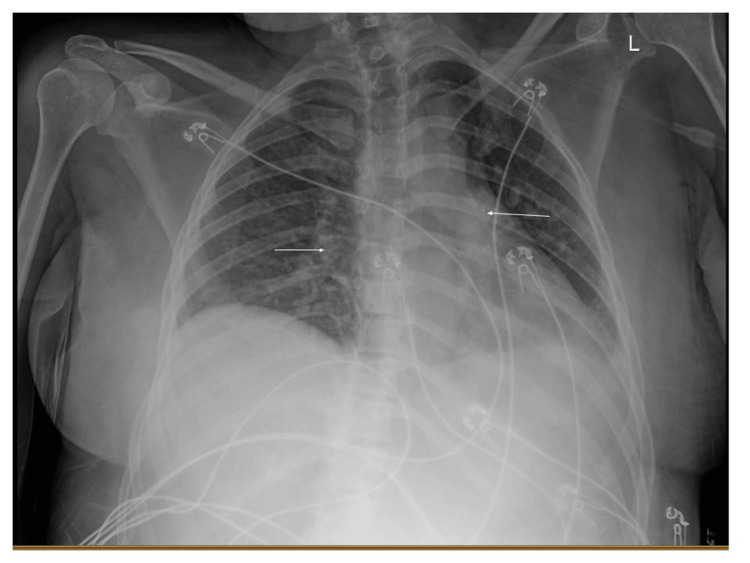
Initial chest X-ray The arrows point to bilateral patchy infiltrates.
